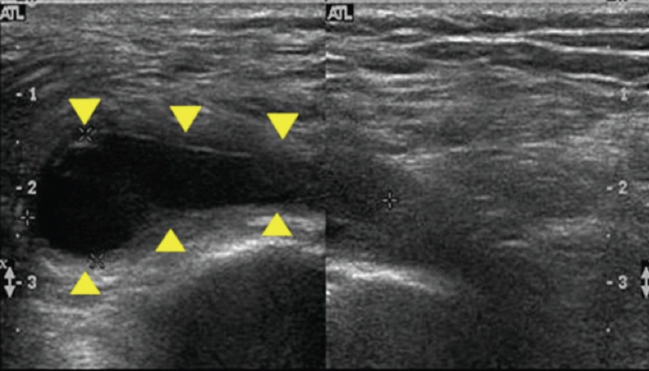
The image of ultra-sonography in a 76-year female shows hypoechoic lesion (arrow head) with acoustic enhancement represents the ischiogluteal bursitis.
